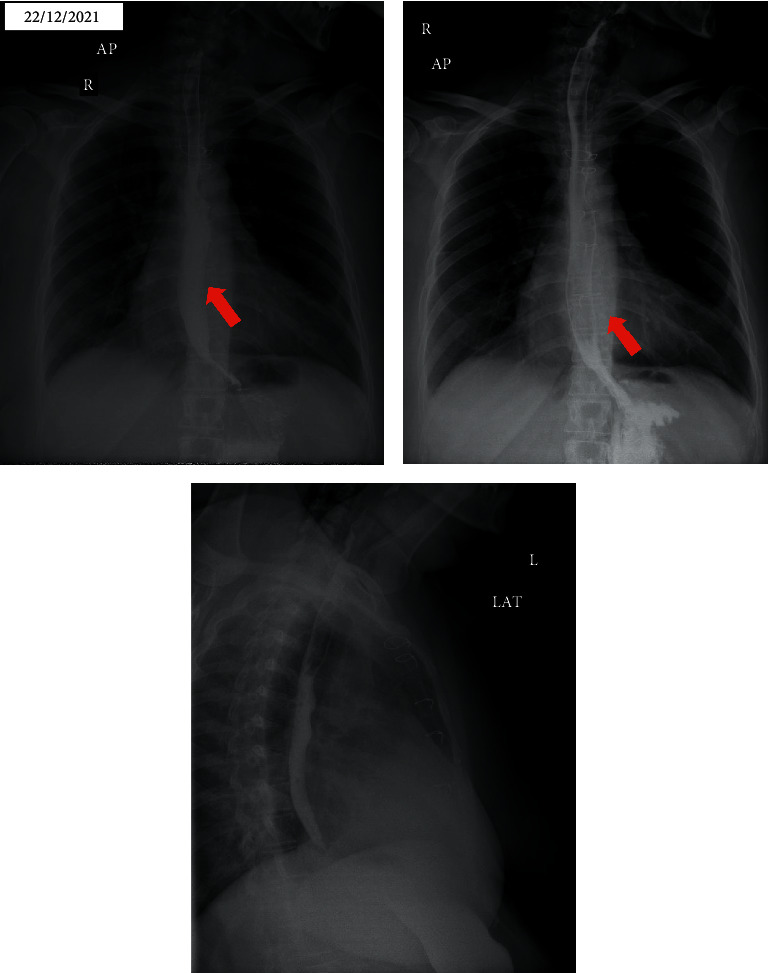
Anteroposterior (AP) and lateral (LAT) chest radiography after barium ingestion showed a mildly dilated and irregular esophageal lumen causing asymmetric wall thickness, especially in anteroposterior projection (red arrows) *(date performed: December 22, 2021)*.
